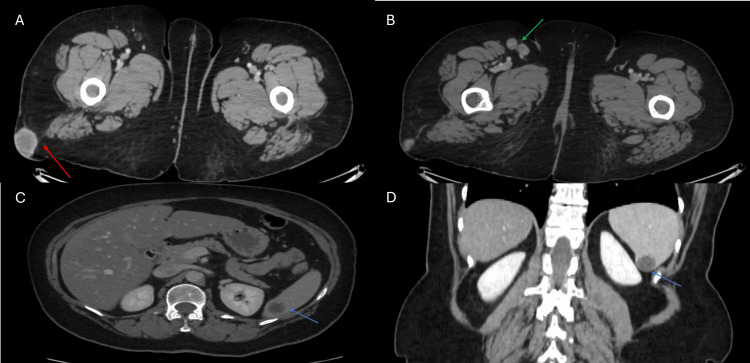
Abdomen and pelvis CT (A, B) Axial and coronal CT images with IV contrast (venous phase): At the lower cuts of the abdomen and pelvis, a CT scan was performed as part of the workup. There is a suspicious enhancing soft tissue lesion in the right subcutaneous gluteal region/proximal thigh, measuring 3 cm (red arrow). Also, noted are two round pathological-looking right inguinal lymph nodes, the largest measuring 1.8 cm x 1.4 cm (green arrow). C, D: A well-defined hypodense lesion showing near fluid density seen at the lower pole of the spleen (blue arrow).
